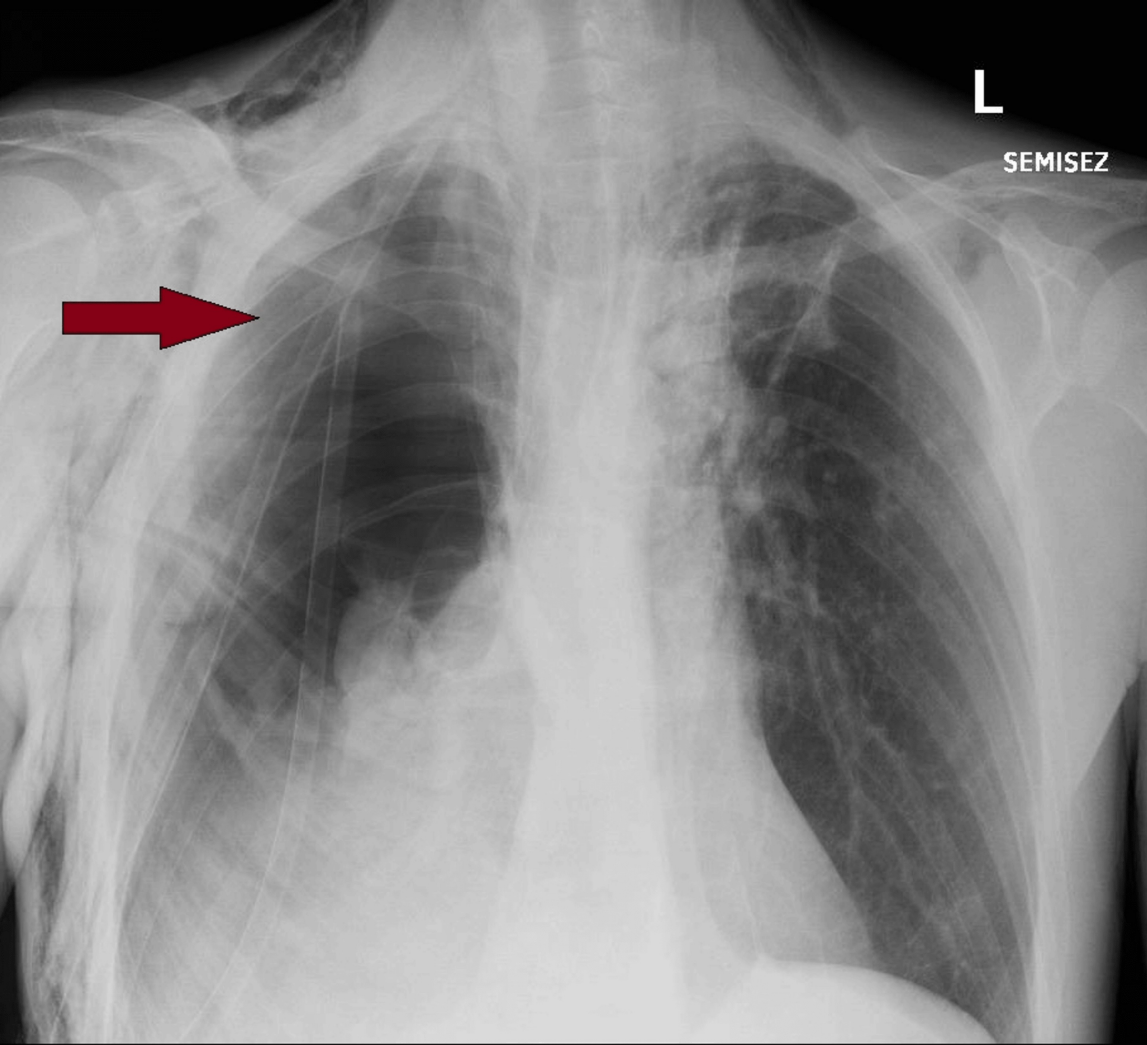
Anterior-posterior view of the chest X-ray on postoperative day 2 – Collapsed lung at the hilum with subcutaneous emphysema - red arrow.
